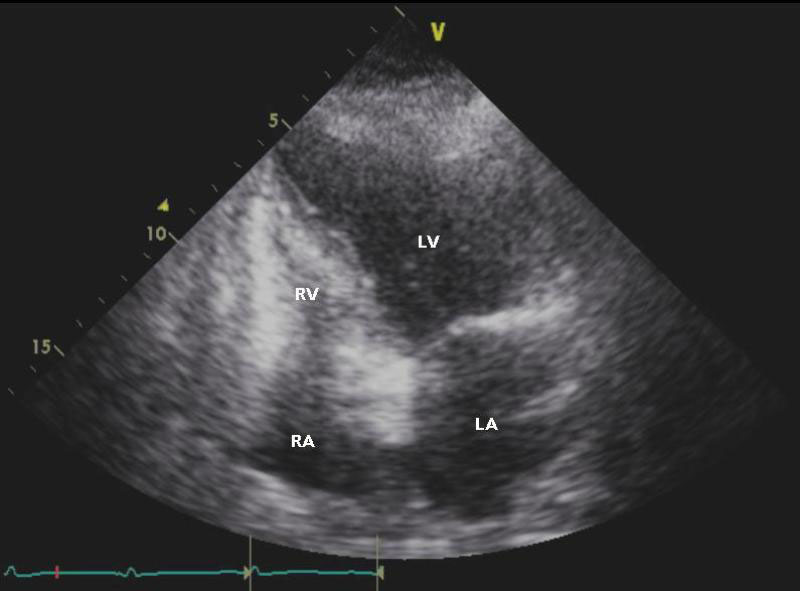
Echocardiogram in systole (left ventricle systolic dysfunction).
